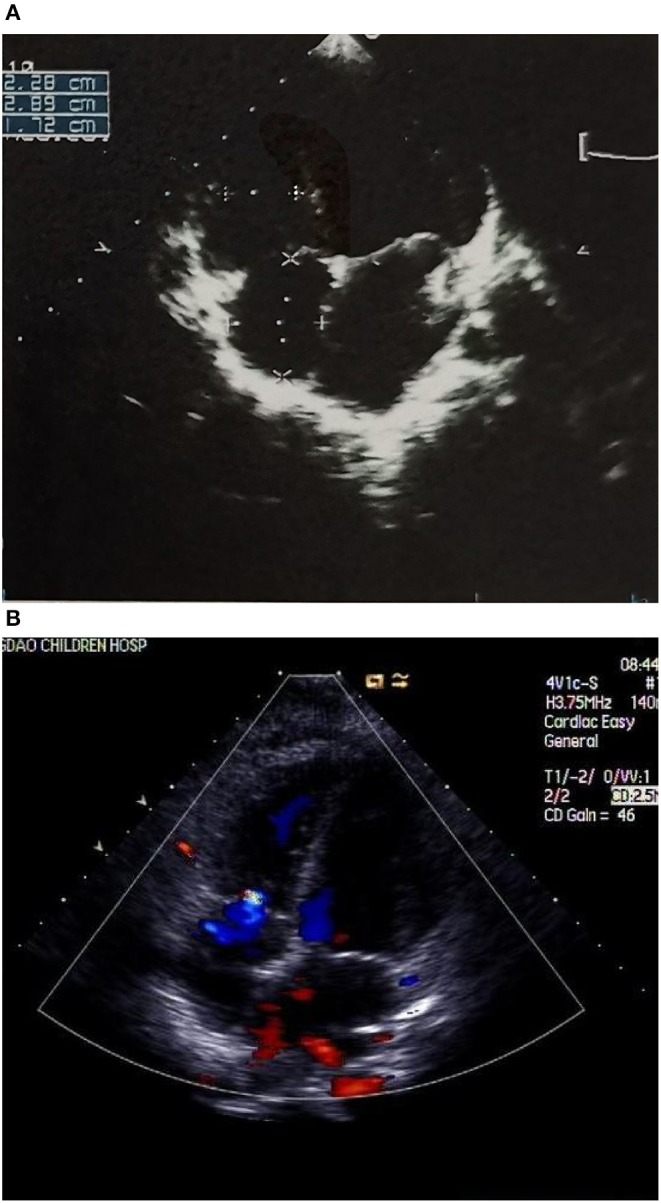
(A) Echocardiograph showing an enlarged left ventricle (LVEDD 35 mm) (B) Echocardiograph showing a relatively normal heart size (LVEDD 45 mm) and a mild tricuspid regurgitation.
